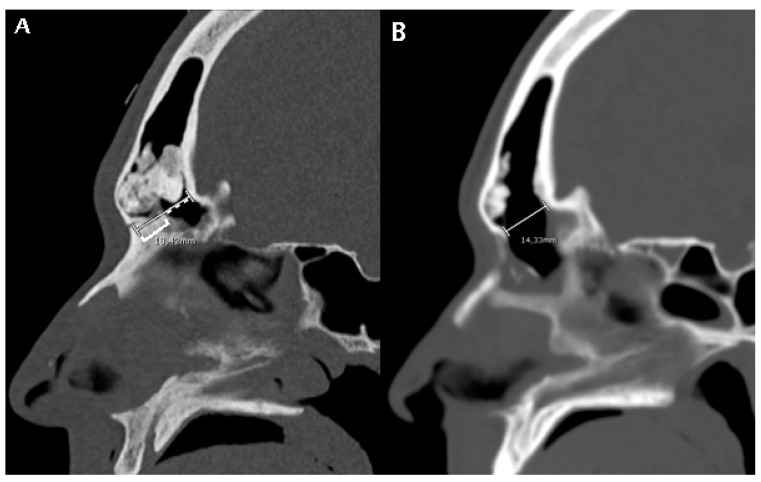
A patient subjected to a DRAF III for relapse of osteoma and fronto-orbital mucocele. (A) shows a pre-operative CT scan where FOAP max (white line) is measured as the sum of the mid-sagittal thickness of the nasal beak (white square bracket) and the mid-sagittal distance between the nasal beak and the most anterior skull base (white dotted line). (B) shows the post-operative CT scan on the same mid-sagittal section. The white line represents the maximum anteroposterior diameter of the frontal sinus neostium after surgery, which we termed obtained diameter (OD, see Figure 5).
